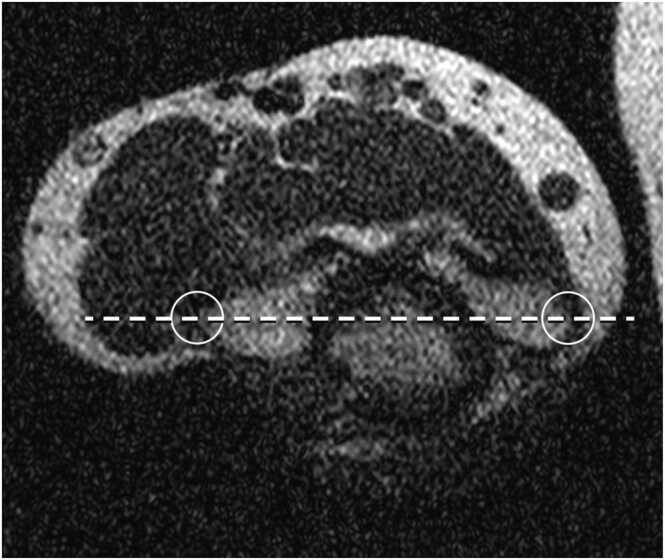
Determination of the epicondylar axis on routine low resolution MRI images.
